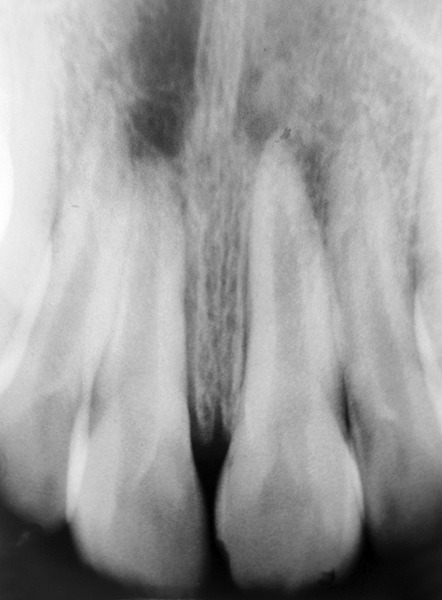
Postoperative periapical radiograph after 6 months showing erupted permanent central incisor
